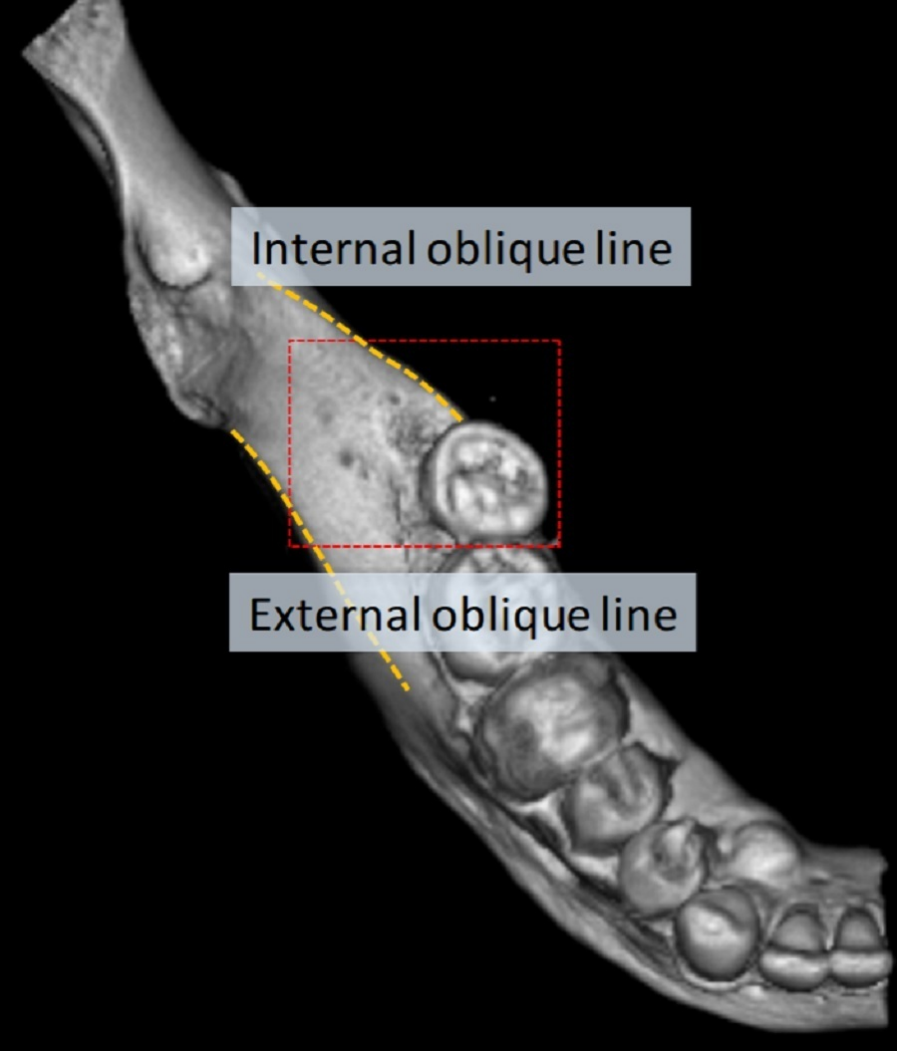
A cone beam computed tomography scan of the right mandible demonstrating triple retromolar foramina posterior to the third molar.
